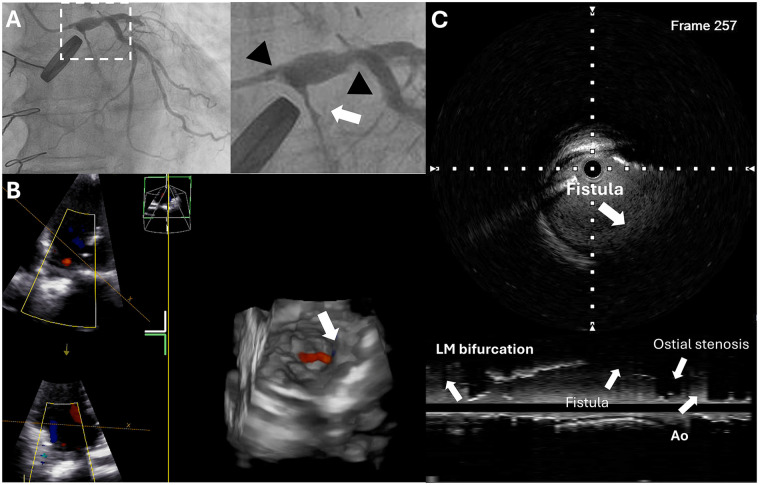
(A) angiographic image and magnification demonstrating the diseased left main (LM) and the presence of a coronarocameral fistula (arrow). Arrowheads indicate the sites of ostial and distal LM stenoses. (B) 3D transthoracic echocardiography (3D TTE) images derived from two non-orthogonal x-plane images showing flow (arrow) entering the left ventricle from a region outside the prosthetic valve or its annulus, anatomically consistent with the course of the LM artery. (C) Intravascular ultrasound (IVUS) image from the left main shaft, demonstrating an opening just distally to the stenosis of the LM ostium. Ao, aorta.
